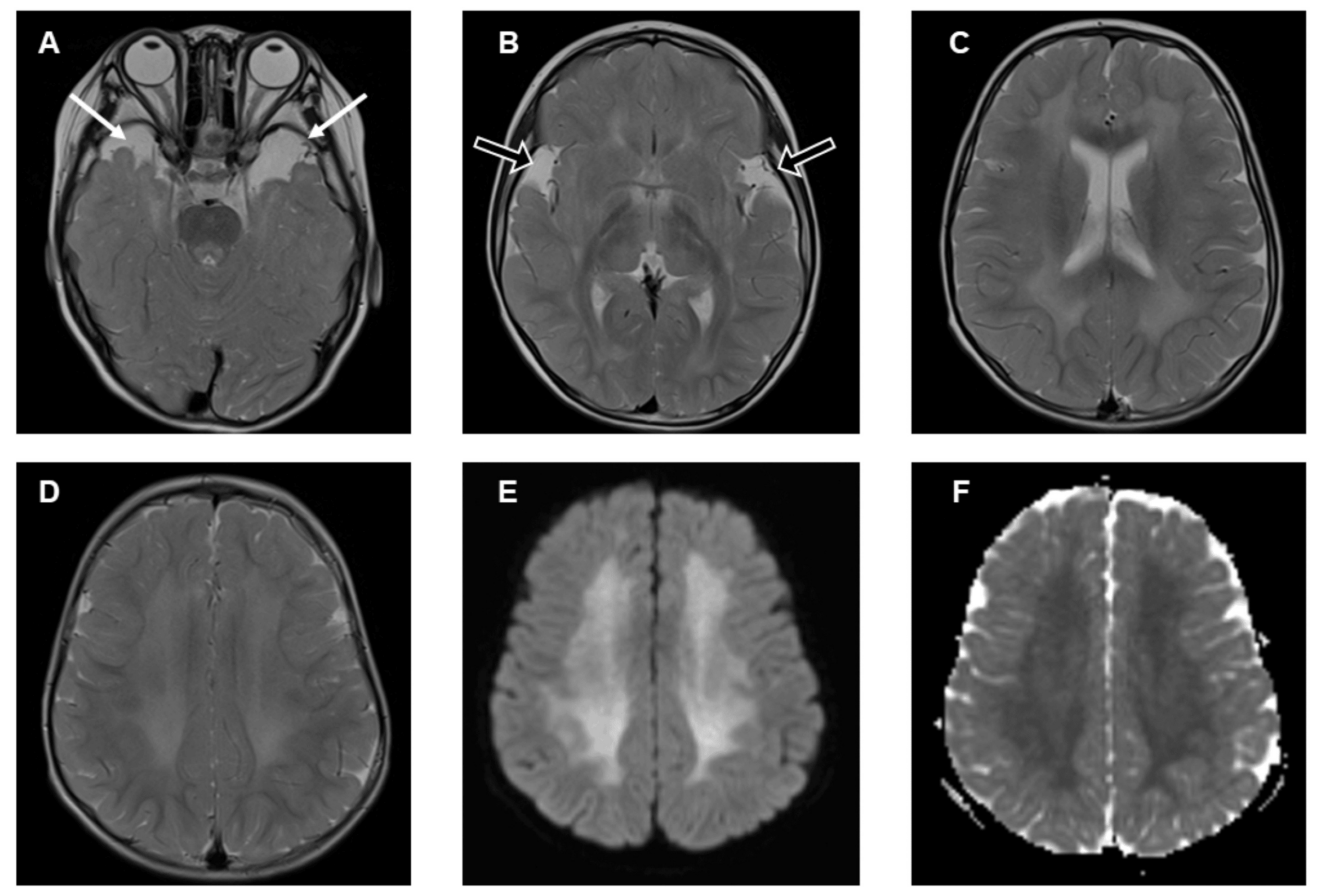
MRI features of the patient at four years old (before surgery) Axial T2-weighted MR images (A-D), a diffusion-weighted image (E), and an apparent diffusion coefficient (ADC) map (F) are shown. Enlarged anterior temporal fossa subarachnoid spaces (white arrows in A) and dilation of the Sylvian fissures (outlined arrows in B) were observed. Diffuse high-intensity signals in T2-weighted images (C, D), corresponding diffusion restriction on the diffusion-weighted image (E), and a drop in the ADC map (F) were observed in the bilateral frontal and temporal white matter. These findings showed almost no change from the MR images taken at birth.
